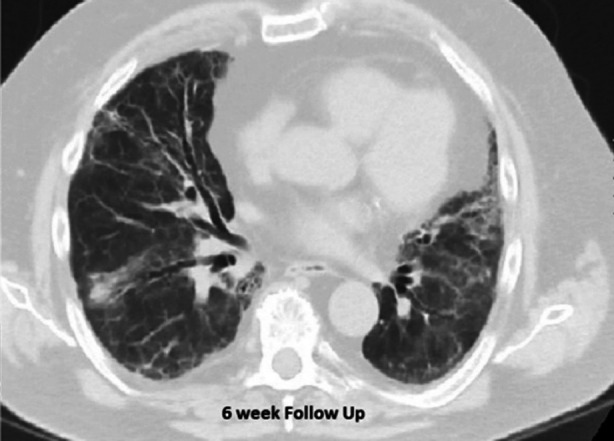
HRCT chest at 6 weeks follow up.
